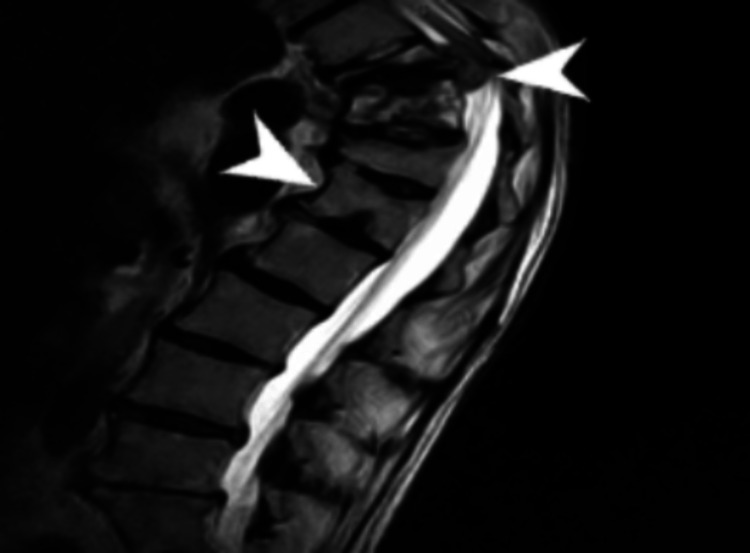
MRI showing acute compression fractures of T10 and T11 Acute compression fractures of T10 and T11 with kyphotic deformity can be seen: 90% height loss at T10, 30% loss at T11. There is also some chronic compression deformity of T12. There is a ventral epidural mass behind the T10 vertebral body causing severe spinal stenosis and compression of the traversing spinal cord MRI: magnetic resonance imaging
